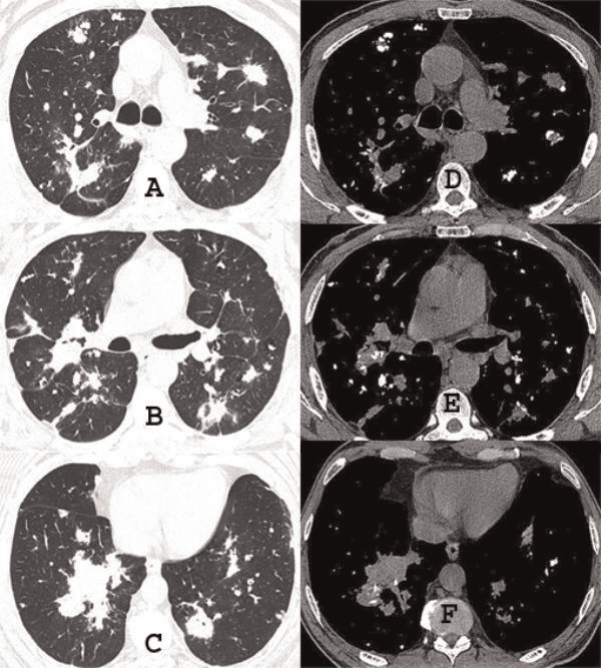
Chest CT obtained with lung window settings (A, B and C), revealing nodules and masses, and irregular contours in both lungs. CT images obtained with mediastinal window settings (C, D and E) show pulmonary lesions permeated by calcifications.
